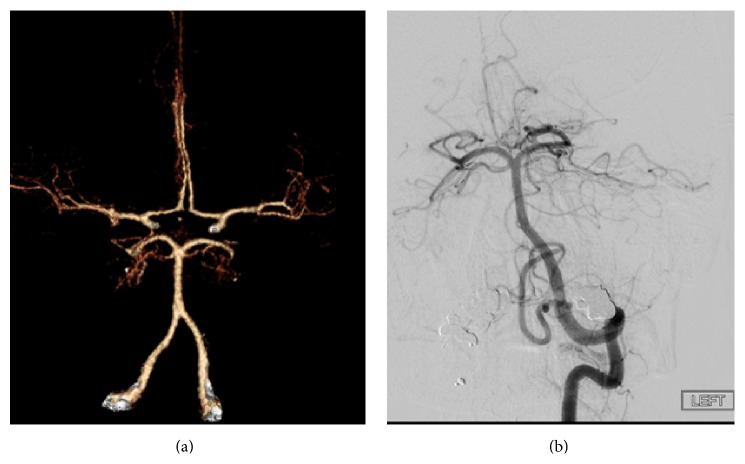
CT angiography (a) and a 4-vessel cerebral arteriogram (b) showed no intracranial aneurysms.
